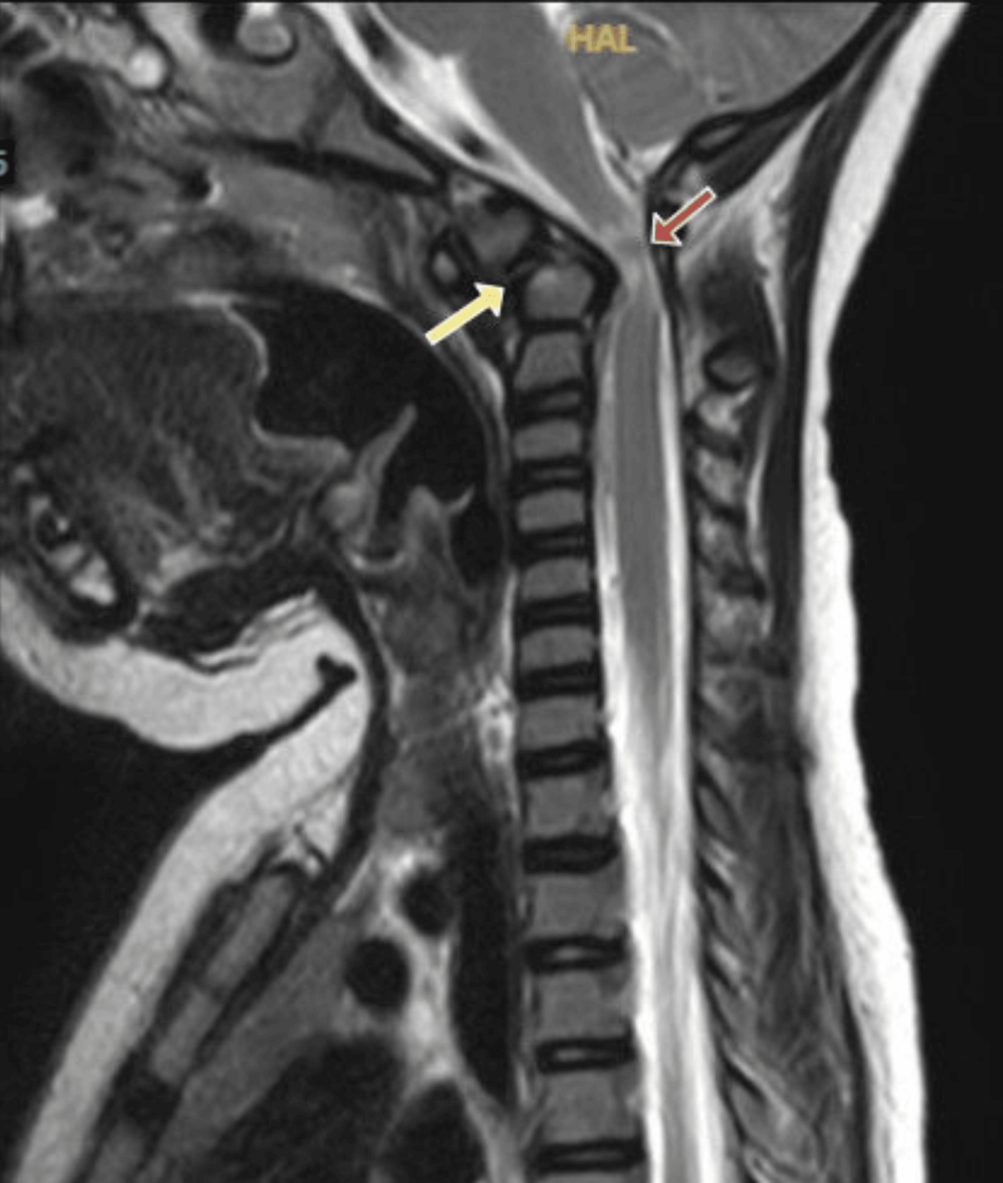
Sagittal T2-weighted MRI of the cervical spine showing cervicomedullary cord compression (red arrow) secondary to anterior subluxation of os odontoideum (yellow arrow).
